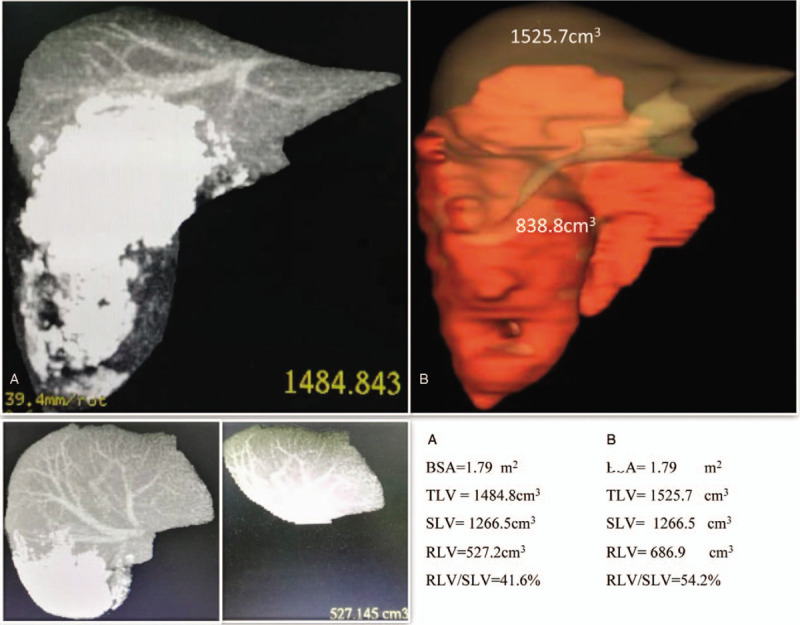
Assessment of resectability and compensation ability of residual liver by CT three-dimensional image postprocessing (A) and 3D visualization technique (B).
